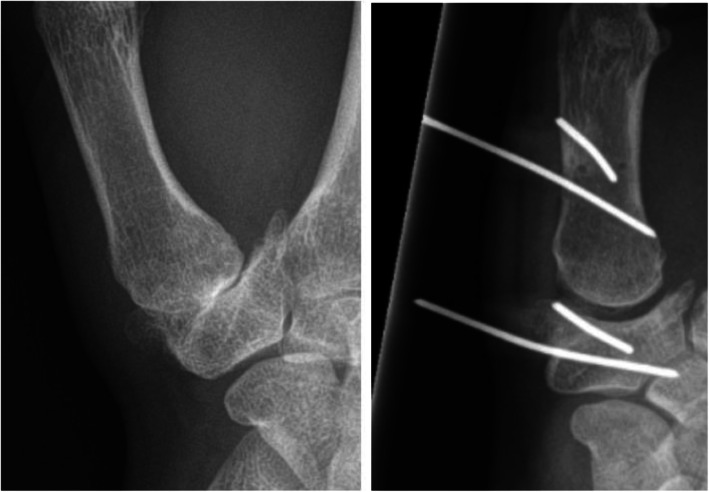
Radiograph of the CMC1 joint before (left) and during distraction (right). Published with permission of the original authors and the Journal of Plastic Surgery and Hand Surgery www.tandfonline.com (reference 19)
